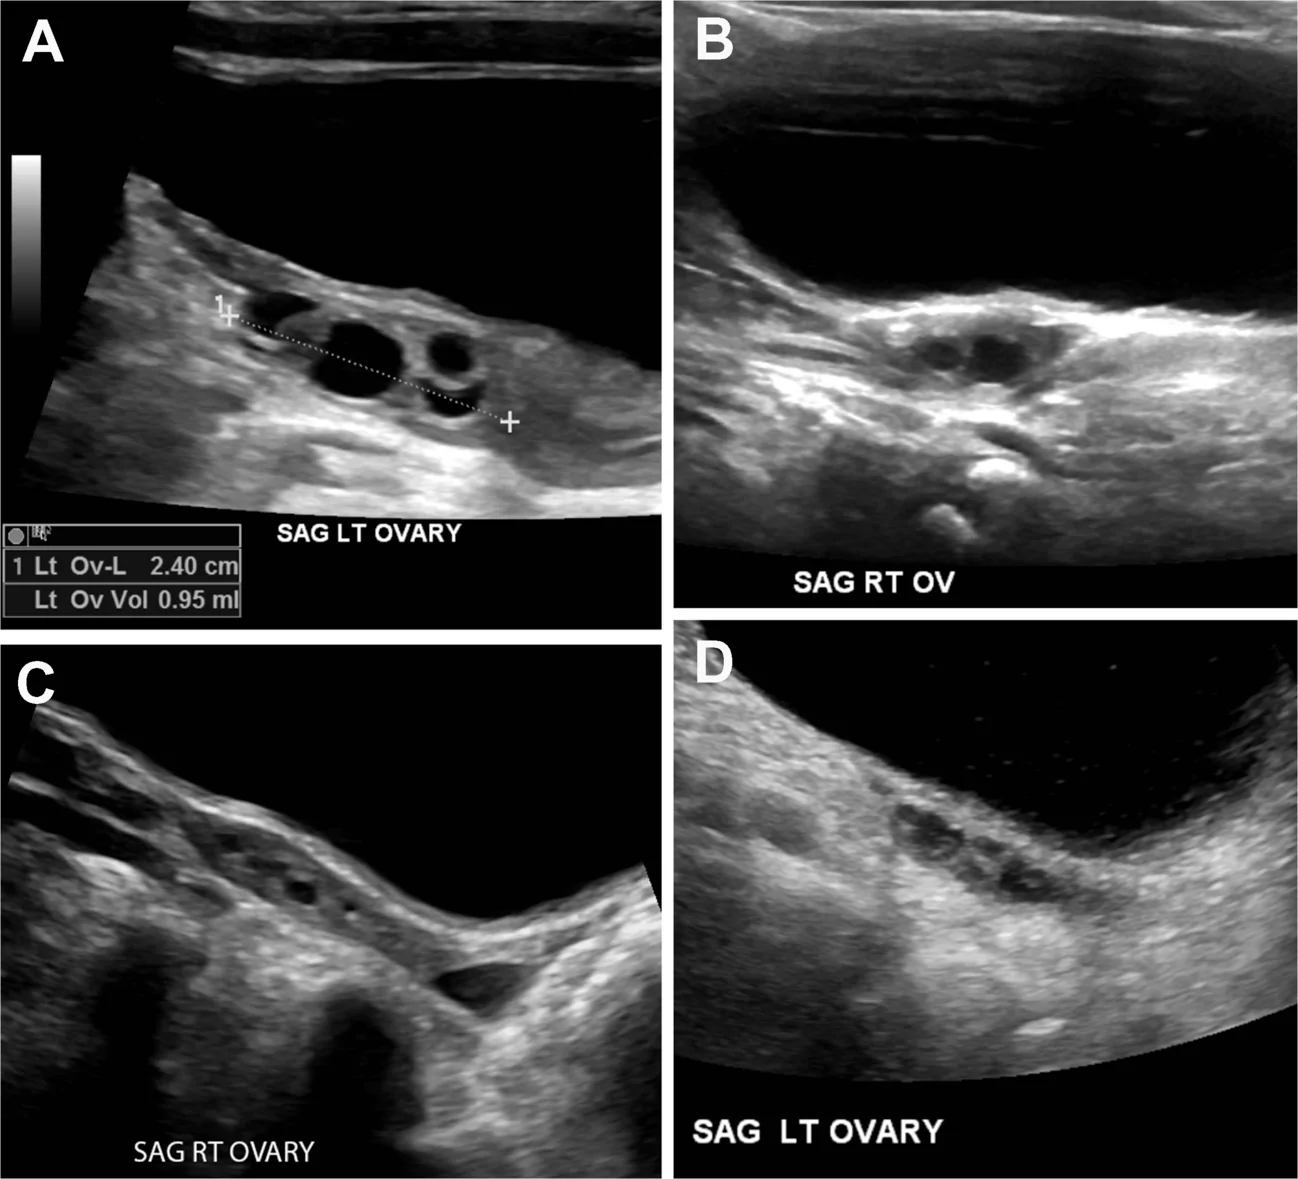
Normal appearance of ovaries in the infant, young child, and postpubertal child. Ultrasound images of normal ovaries show a rounded to oblong appearance of the ovary with several small follicles at the following ages: (a) 6 months (0.95 mL); (b) 9 months (0.45 mL); (c) 4 years (2.7 mL); (d) 9 years (4.5 mL)
